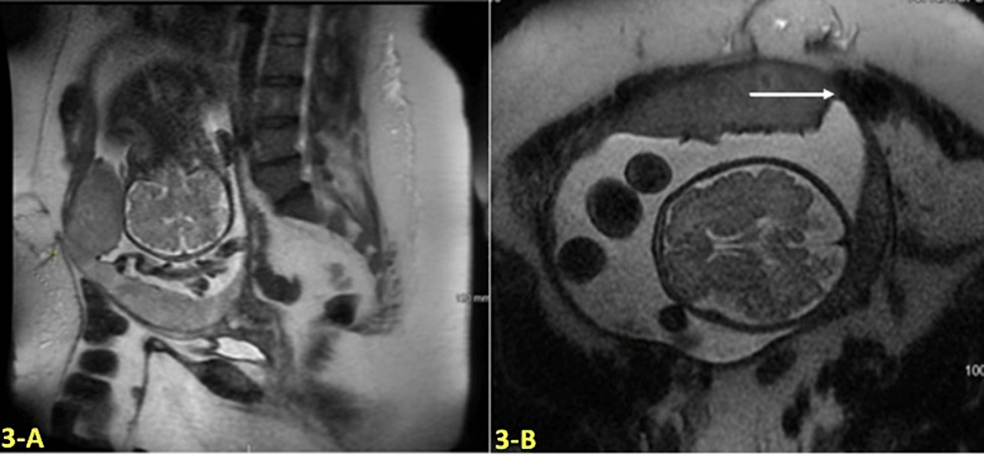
Sagittal single-shot fast spin echo (SSFSE) (A) and Axial T2 weighted SSFSE (B) Magnetic resonance imaging (MRI) findings at 33 weeks gestational age (GA) A and B: Magnetic resonance imaging (MRI) at 33 weeks gestational age (GA) showing a focal bulge of the uterus now in contact with the abdominal wall and a loop of the colon with loss of its normal fat plane indicated by the white arrow.
